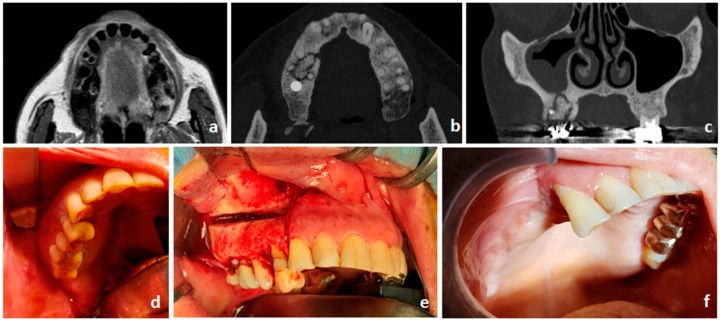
Clinical aspect of non-exposed bone variant of ONJ. The same patient from Figure 3 did not complete the recommended periodontal evaluation of dental elements of the upper right jaw. She developed a recurrent upper dental abscess, with swelling of the right cheek. This is a clinical manifestation of the non-exposed bone variant of ONJ. (a) Preoperative magnetic resonance imaging (MRI) showing high signal intensity of periodontal space in the right upper jaw. (b) Computed tomography (CT) scan in axial view showed large areas of increased medullary bone density of the upper dento-alveolar process. Bone sequestration was present close to periodontal disease. (c) CT scan in coronal view showed large areas of increased medullary bone density of the upper dento-alveolar process. Bone sequestration was present close to periodontal disease. Upper jaw involvement is related to a maxillary sinusitis. (d) Preoperative intraoral view with absence of exposed necrotic bone (unexposed variant of ONJ). (e) Intraoperative view of right partial upper maxillectomy. (f). Postoperative healed intraoral view. Primary closure of the surgical site was obtained by Bichat buccal fat pad transfer and mobilization of a muco-periosteal flap.
